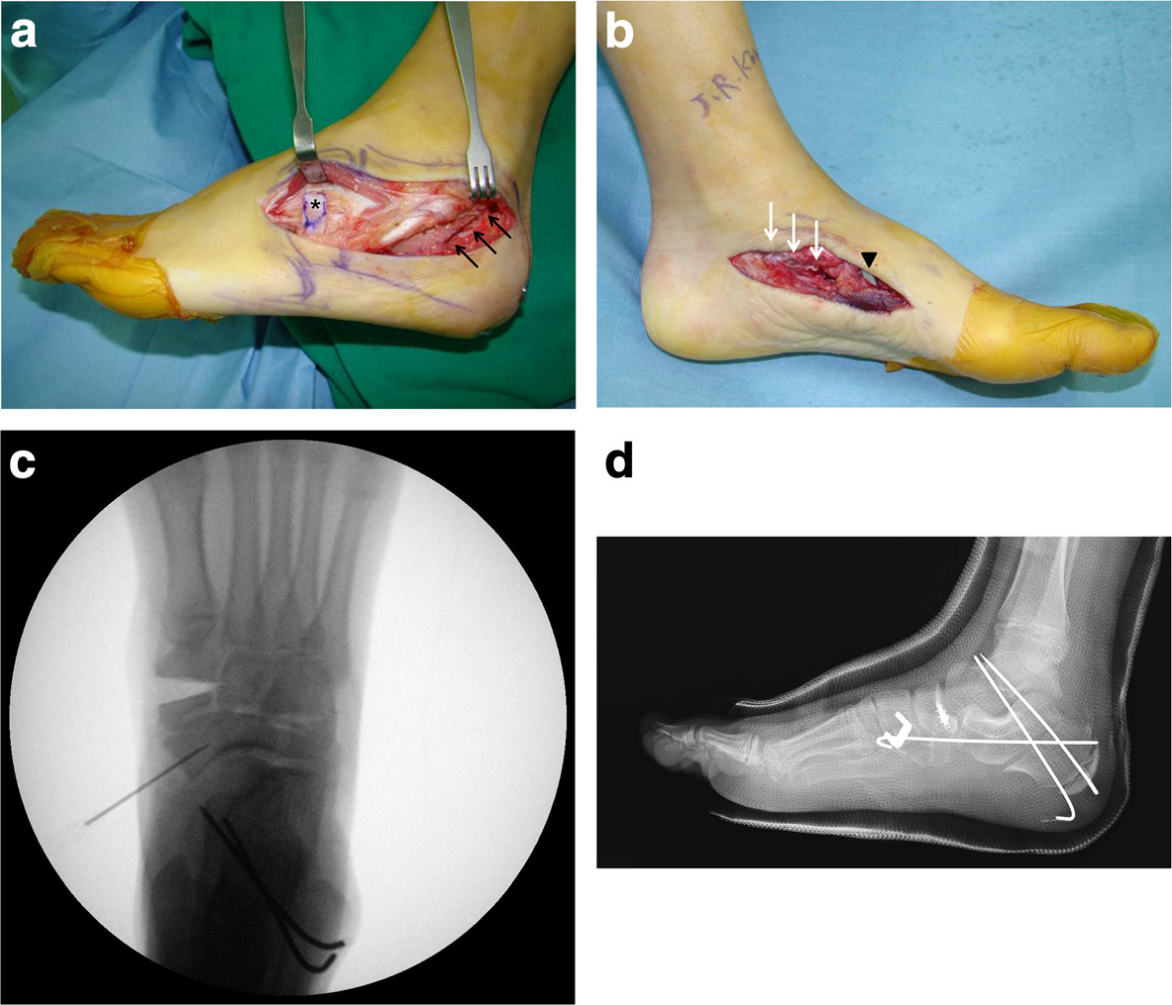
Triple C combined with the modified Kidner procedure. (a) Lateral view of the foot showing calcaneal osteotomy (black arrows) and insertion of the opening wedge graft from the cuneiform into the cuboid (asterisk). (b) Medial view of the foot showing plantar flexion and pronation of the foot achieved with closing wedge osteotomy of the medial cuneiform (arrowhead), providing reconstruction of the longitudinal arch, and the TPT was advanced and attached to the navicular with the two no. 2 braided nonabsorbable sutures attached to the anchor (white arrows). (c) Positioning of the anchor was confirmed under intraoperative fluoroscopy. (d) Immediate postoperative radiography after combined triple C and modified Kidner procedure.
